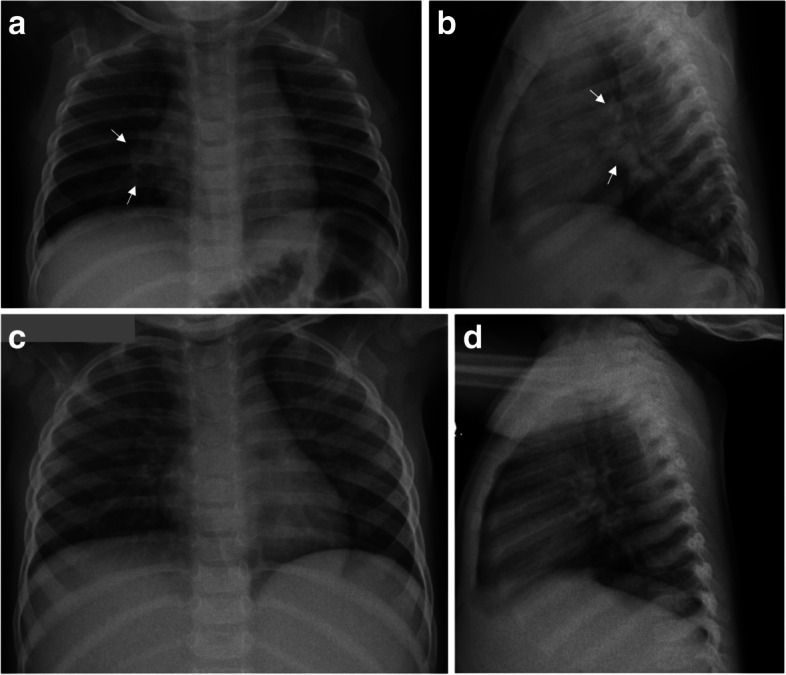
Normal and consistent with tuberculosis chest radiographs from children household contact of patients with tuberculosis. a,b Anteroposterior (a) and lateral (b) chest radiograph consistent with active tuberculosis in a 10-month-old boy. Round opacity parahiliary right suggestive of lymphadenopathy. c,d Normal anteroposterior (c) and lateral (d) chest radiograph in a 15-month-old girl
